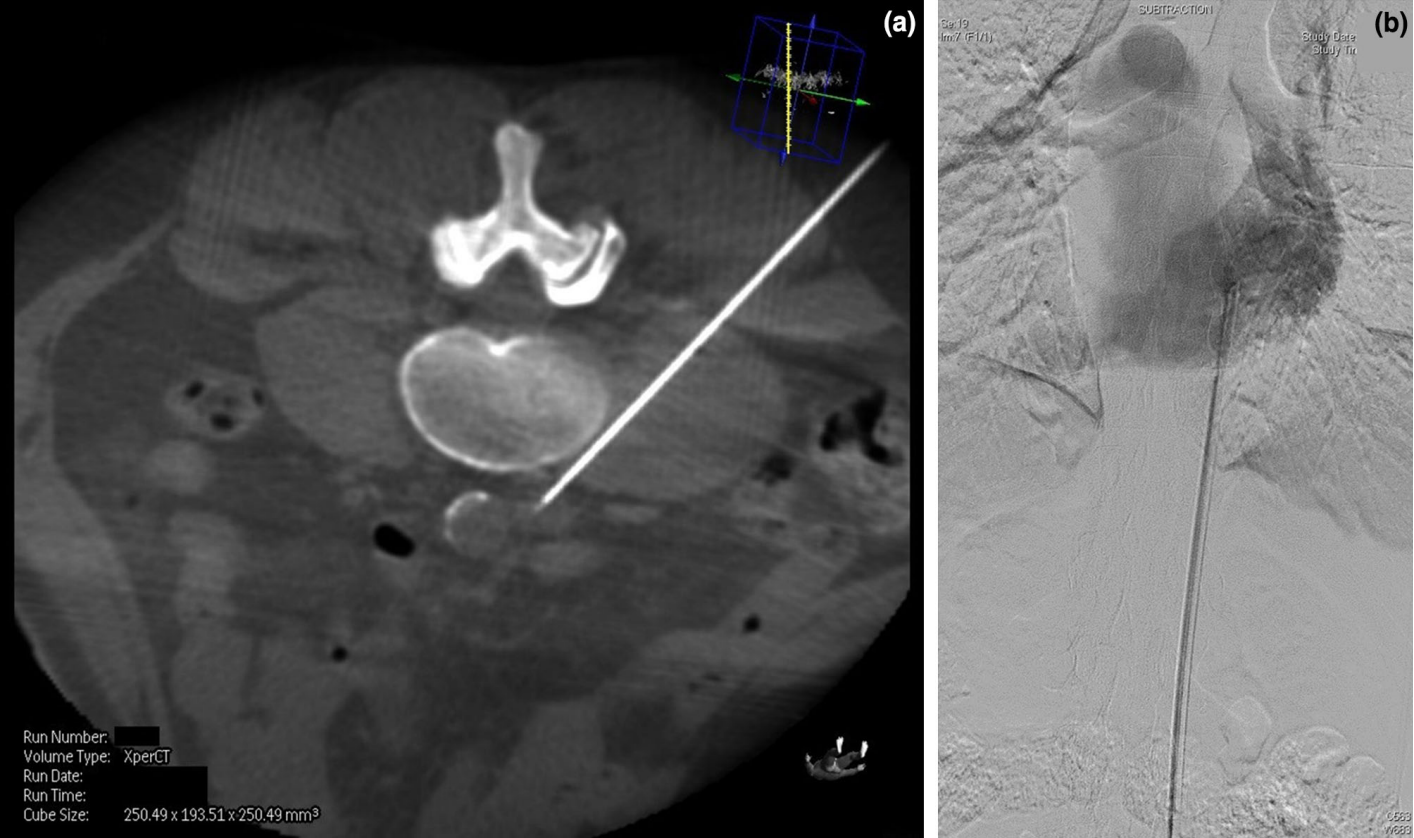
(a) Single cone-beam CT image taken during placement of a translumbar vena cava dialysis line in a patient with no further peripheral vascular access options. The image was used to safely and accurately puncture a stenotic inferior vena cava (IVC) *via* the tranlumbar route. The image shows the needle is the correct position with the tip in the IVC. (b) The tunneled catheter was subsequently placed using the Seldinger technique. The tip of the catheter can be seen adequately positioned within the right atrium on this single DSA image.
